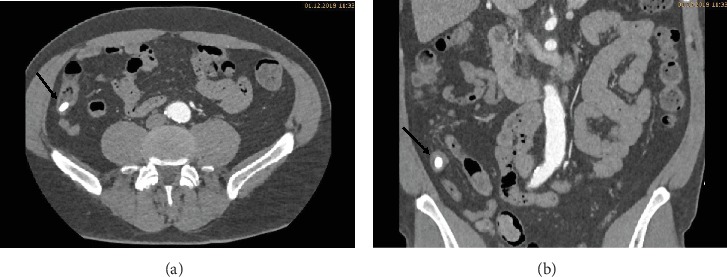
(a, b) Transversal and coronal slides of the abdominal CT in the arterial phase: a sharply demarcated structure in the small bowel is seen.
